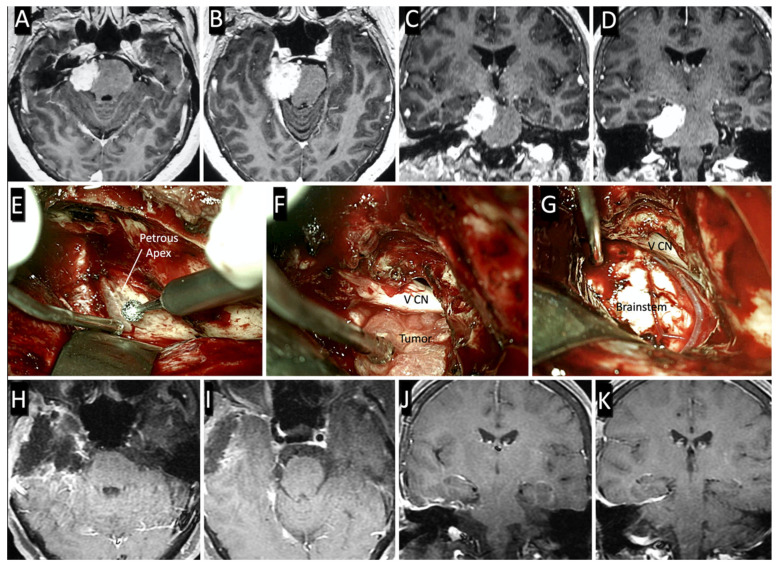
Preoperative axial (A,B) and coronal (C,D) T1-weighed contrast-enhanced MRI showing the petroclival meningioma. (E–G) Main steps of the anterior petrosal approach. (E) Peeling of the middle fossa dura and exposure of the petrous apex. (F) Intradural tumor exposure and initial debulking. (G) Surgical field after tumor resection and visualization of the lateral aspect of the pons. Postoperative axial (H,I) and coronal (J,K) contrast-enhanced T1-weighed MRI showing the gross total resection of the tumor (Simpson grade I).
